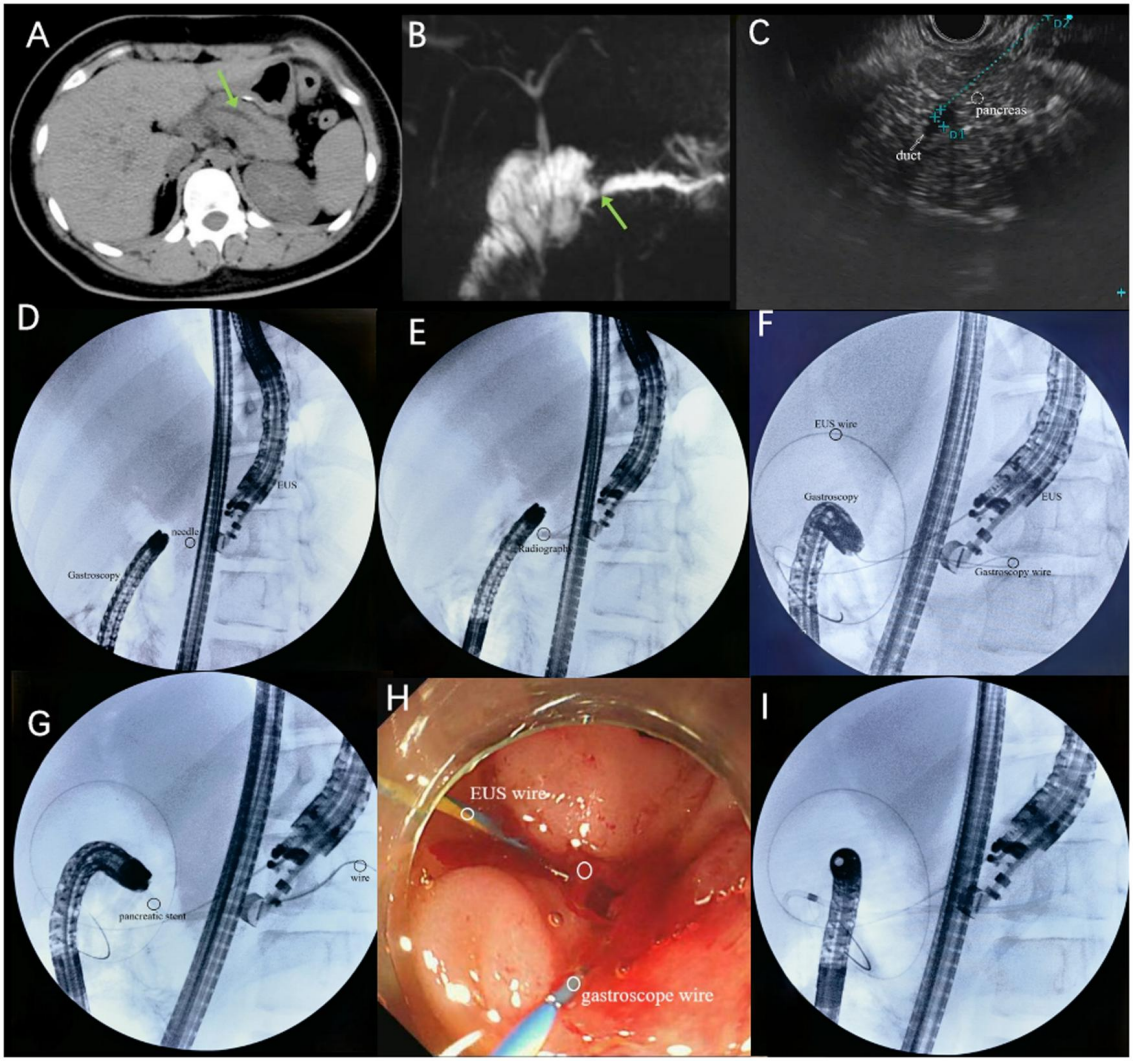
EUS-guided pancreatic duct drainage with gastroscopy for pediatric pancreaticojejunostomy stricture. (A) CT shows residual pancreatic tissue with dilated pancreatic ducts. (B) Stricture at the pancreaticojejunal junction is shown in magnetic resonance cholangiopancreatography. (C) EUS measured the diameter of the pancreatic duct and the distance between the pancreatic duct and the puncture needle. (D) Puncturing the pancreatic duct under EUS (black circle). (E) The pancreatic duct could be seen when the iohexol was placed along the puncture needle after EUS-PD. (F) A guide wire is anterograde placement by EUS-PD and then into the intestinal lumen, the other guide wire is retrograde placement by gastroscopy (black circles shows wires). (G) Placement of pancreatic stents along the gastroscopy wire. (H) EUS-PD anterograde guide wire and gastroscopy retrograde guide wire (white circle). (I) Complete stent placement.
